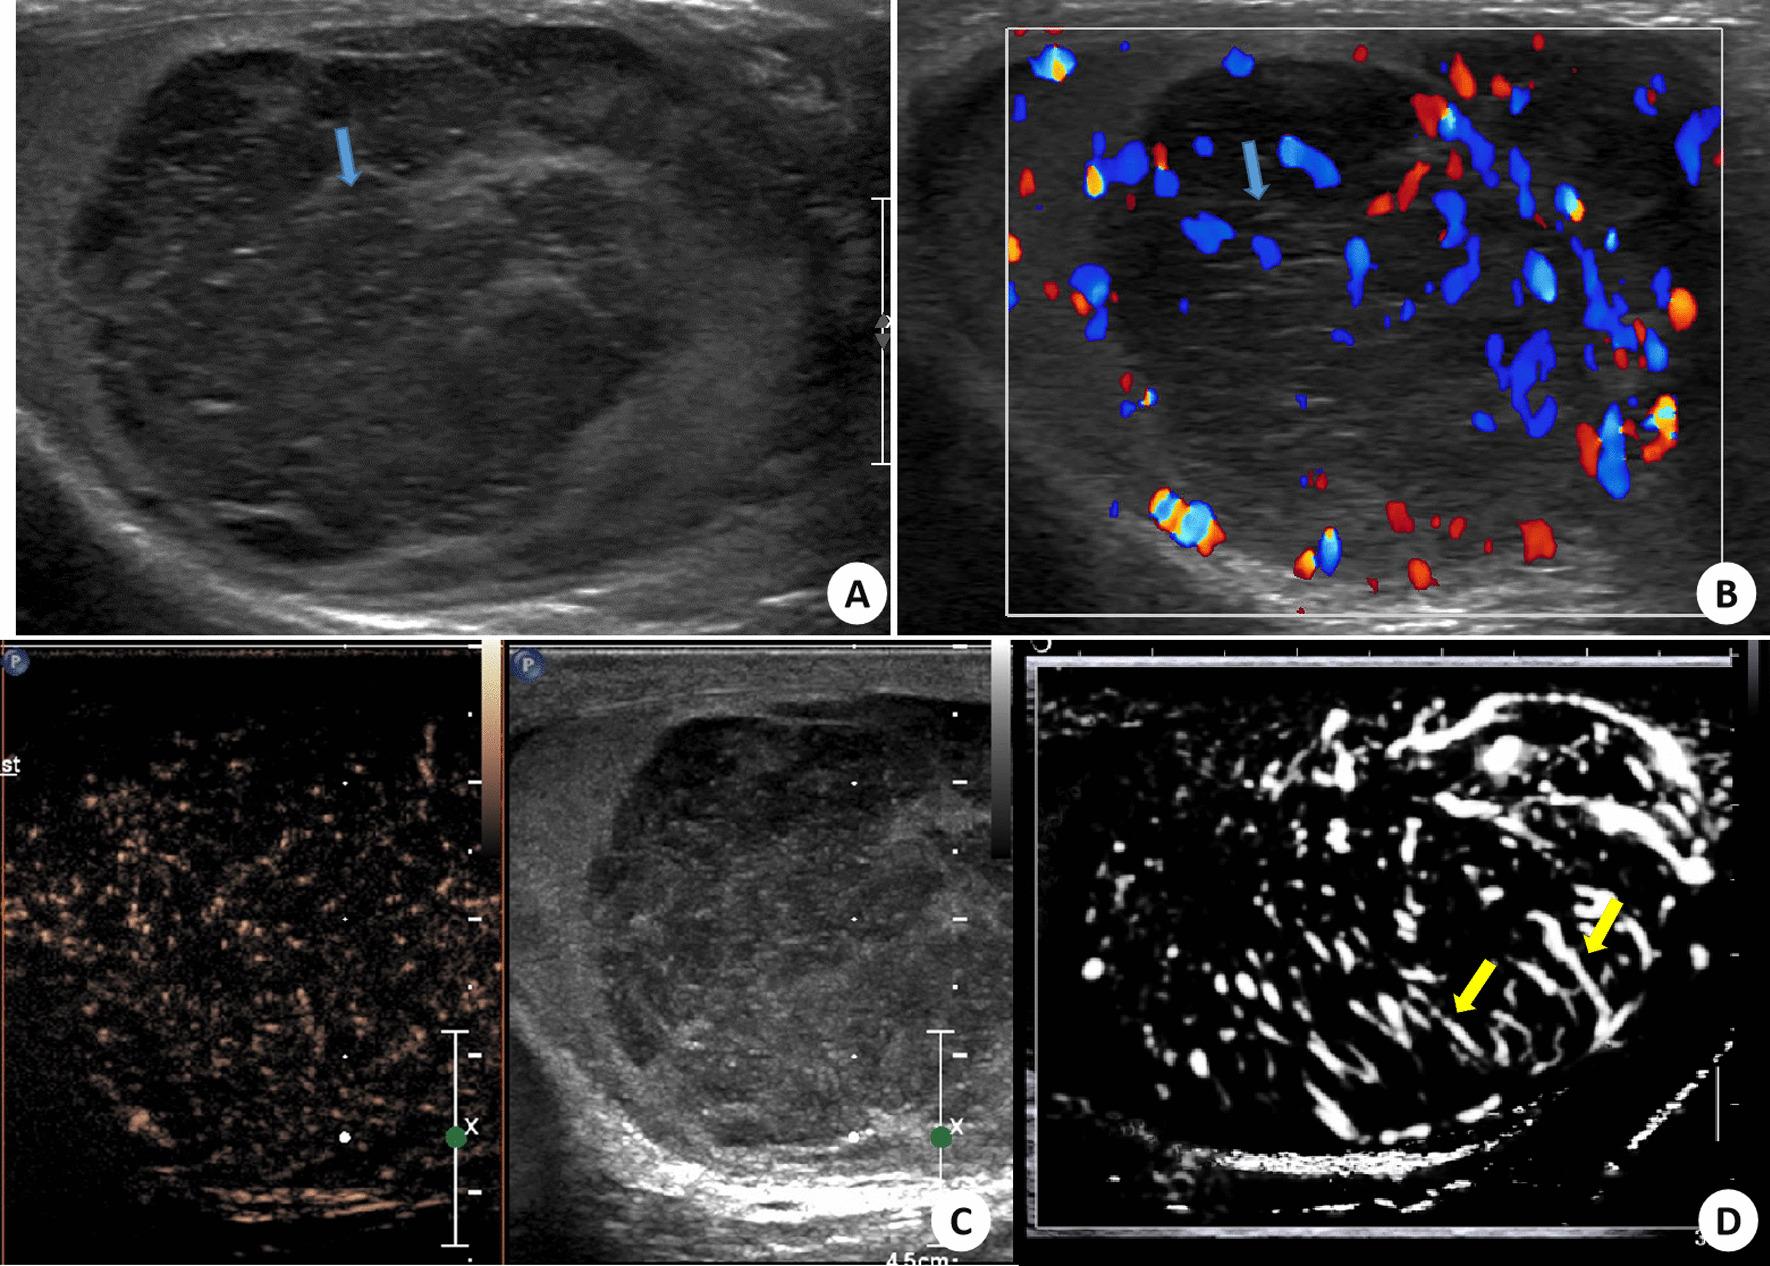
A diffuse large B-cell lymphoma of right testis in a 46-year-old patient diagnosed after orchiectomy. Blue arrow indicated the linear echogenic strands in grey-scale US (A) and CDFI (B) images. The increased enhancement with enlarged range was also seen in CEUS image (C). Yellow arrows (D) indicated the straight and parallel vascular sign on microvascular ultrasonographic image
